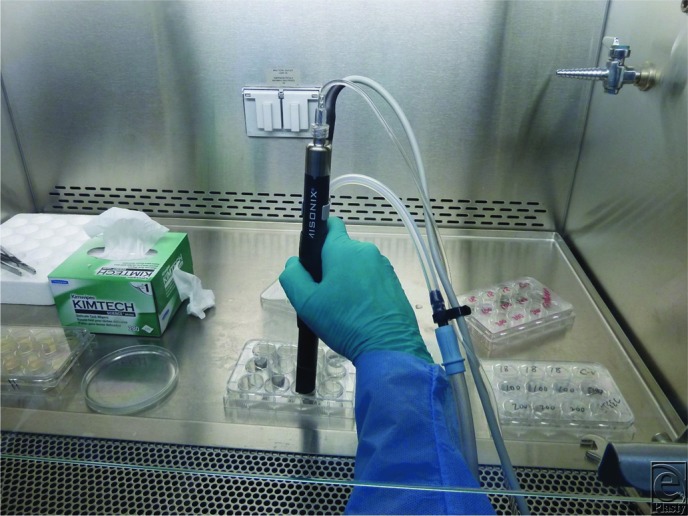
Experimental setup. The study was conducted under a hood. The direct-contact low-frequency ultrasound device was placed 2 mm above each disc for 10 seconds.
